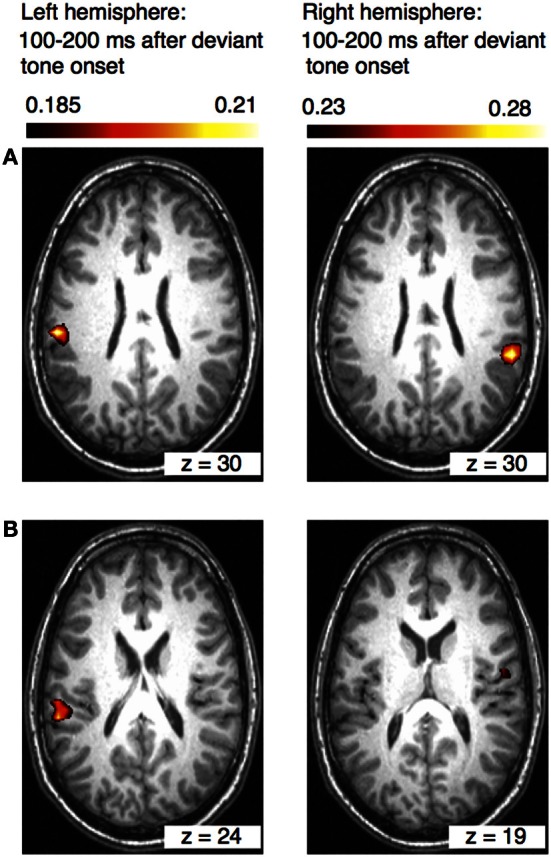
Axial views of the left and right hemispheres (overlaid on an individual anatomical MRI) showing significant activations (pseudo-*T*-values, significance level 5%) of the musically elicited mismatch negativity within a time window of 100–200 ms after the occurrence of a rhythmic deviation. Panel (A) shows activation within the left and right inferior parietal lobule (IPL) (MNI coordinates left: *x* = −58, *y* = −27, *z* = 30; right: *x* = 59, *y* = −41, *z* = 30). Panel (B) shows activation within the left superior temporal gyrus (STG) and in the right insula (MNI coordinates left: *x* = −60, *y* = −34, *z* = 24; right: *x* = 59, *y* = 41, *z* = 19).
